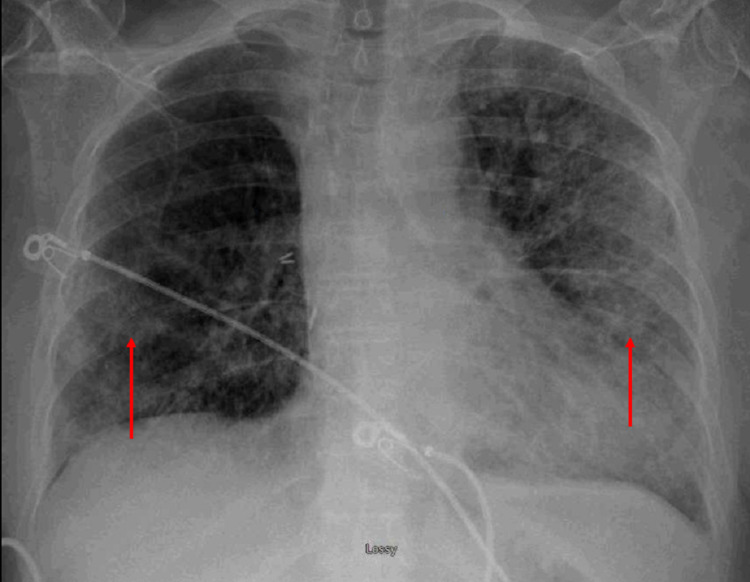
Portable chest X-ray revealed bilateral hazy airspace opacities (red arrows).
